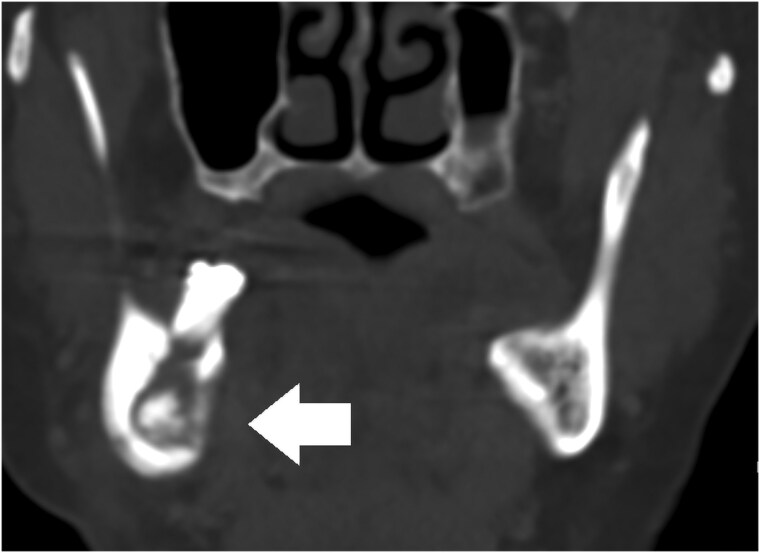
Computed tomography scan (coronal view) from 2023 showing an intraosseous lesion of the right mandible. Image courtesy of the Department of Radiology, Nordland Hospital Trust.
